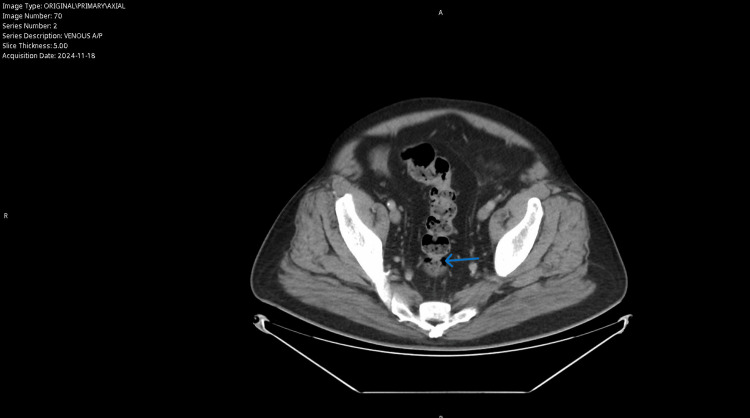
The patient’s abdominal CT scan, showing evidence of the micro-perforation of his sigmoid colon as designated by the arrow
